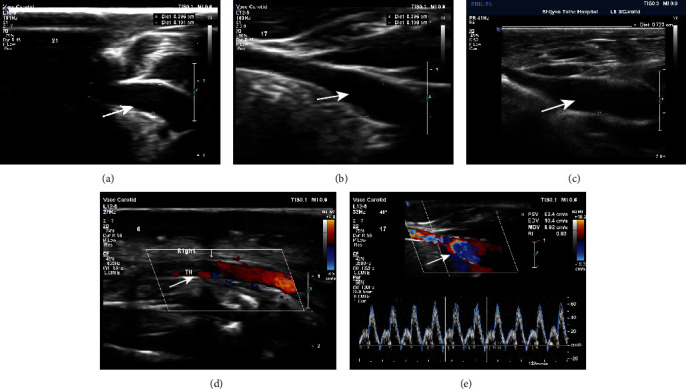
Color Doppler ultrasound demonstration of aneurysm creation. Samples were checked by color Doppler ultrasound. (a–c) B-mode: used to measure diameter. (a–c) refer to Group A, Group B, and Group C. Dist A = 0.396 cm, Dist B = 0.396 cm, and Dist C = 0.732 cm. (d) Doppler was used to measure arterial blood flow, TM pointed to by the arrow is the thrombus, and the red shows the blood movement. (e) Doppler was used to visualize flow inside aneurysm. PSV = 62.4 cm/s, EDV = 10.4 cm/s, and MDV = −8.92 cm/s.
